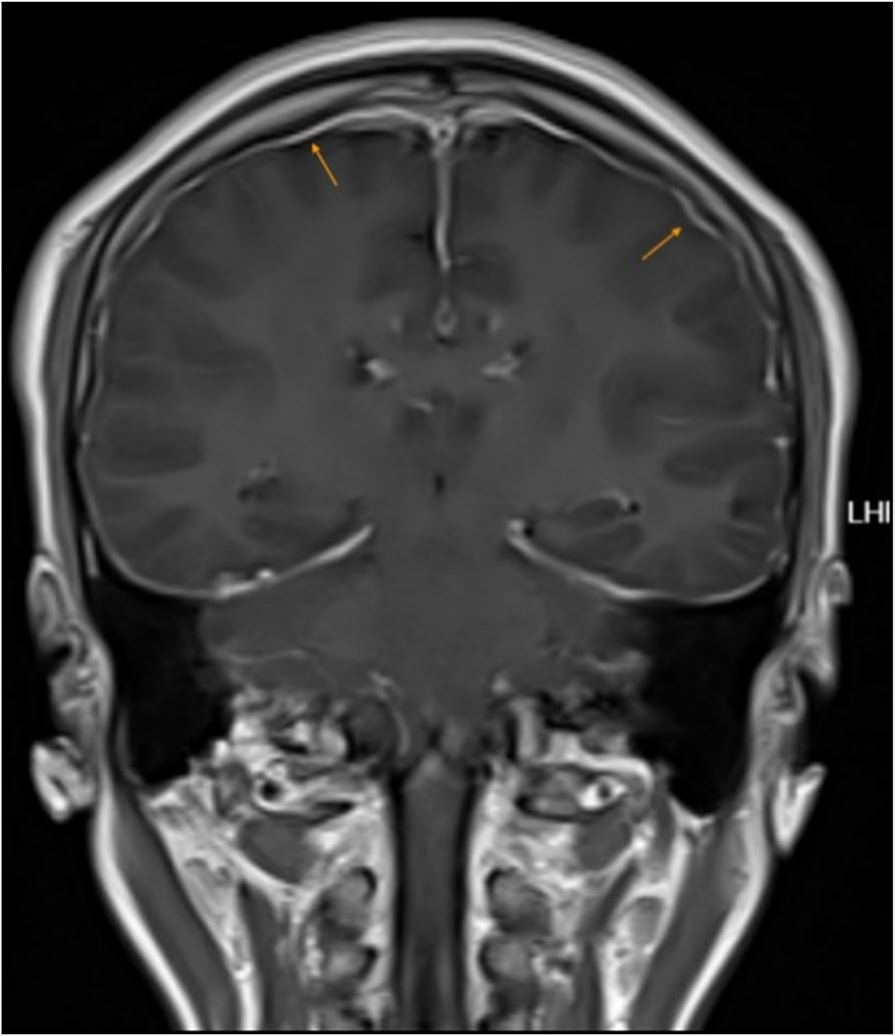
Contrast-enhanced brain MRI demonstrating smooth diffuse pachymeningeal enhancement, which was consistent with intracranial hypotension
